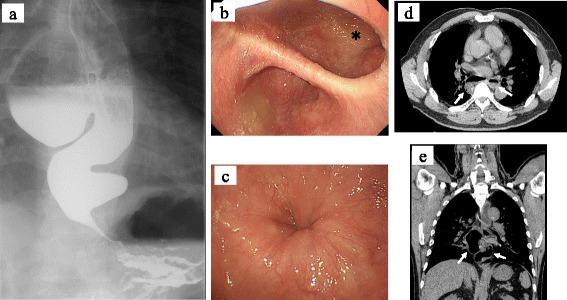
Preoperative imaging findings. a An upper gastrointestinal contrast study demonstrates dilatation of the esophagus and epiphrenic diverticula. b Upper gastrointestinal endoscopy shows a huge diverticulum in the lower esophagus (*asterisk*). c Endoscopic examination showed that the rosette-like esophageal folds appeared in the lower esophagus. d, e Chest computed tomography demonstrates dilatation of the esophagus and the epiphrenic diverticula of 50 × 40 mm and 25 × 25 mm in size. *Arrows* indicate the diverticula
